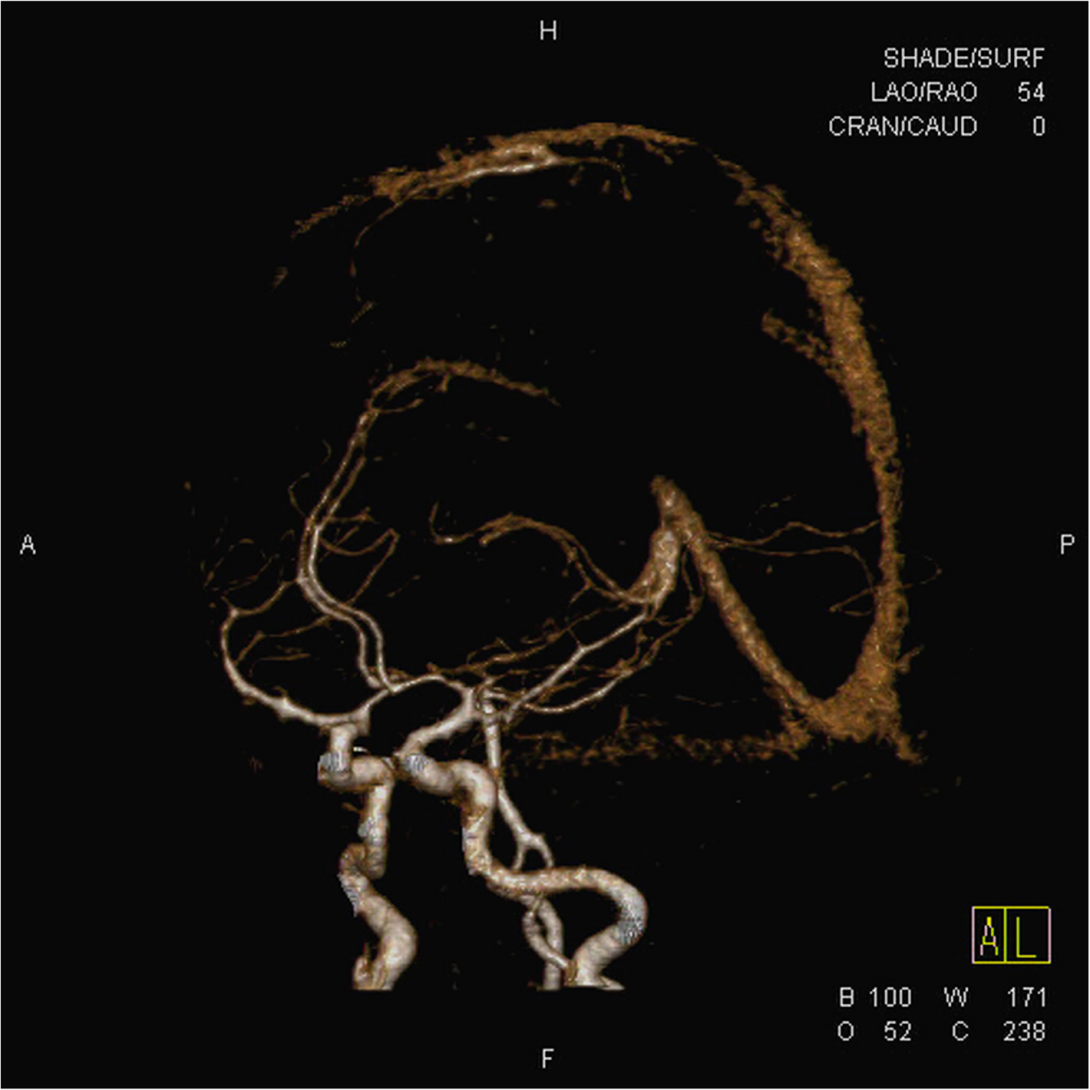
MRI image showed superior sagittal sinus thrombosis with acute infarct and mild subarachnoid haemorrhage
